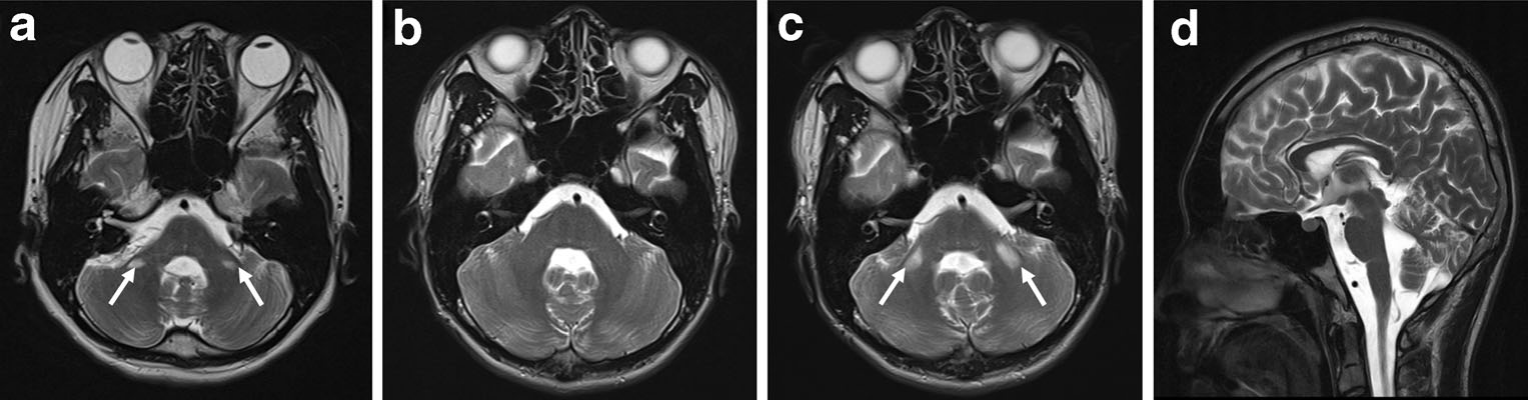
Brain MRI in patient II-1 (a) and patient II-2 (b–d). a High-intensity lesions of the bilateral middle cerebellar peduncle (*arrows*) in T2-weighted imaging (patient II-1; 20 years of age). b At 17 years of age, no high-intensity lesions were found in the middle cerebellar peduncle of patient II-2. c However, a follow-up brain MRI 3 years later revealed bilateral middle cerebellar peduncle lesions (*arrows*). d The bilateral cerebellum and brainstem were atrophied, with prominent cerebellopontine cisternal spaces, a widened fourth ventricle, and enlarged cerebellar cortical sulci were observed in patient II-2 at 20 years of age
